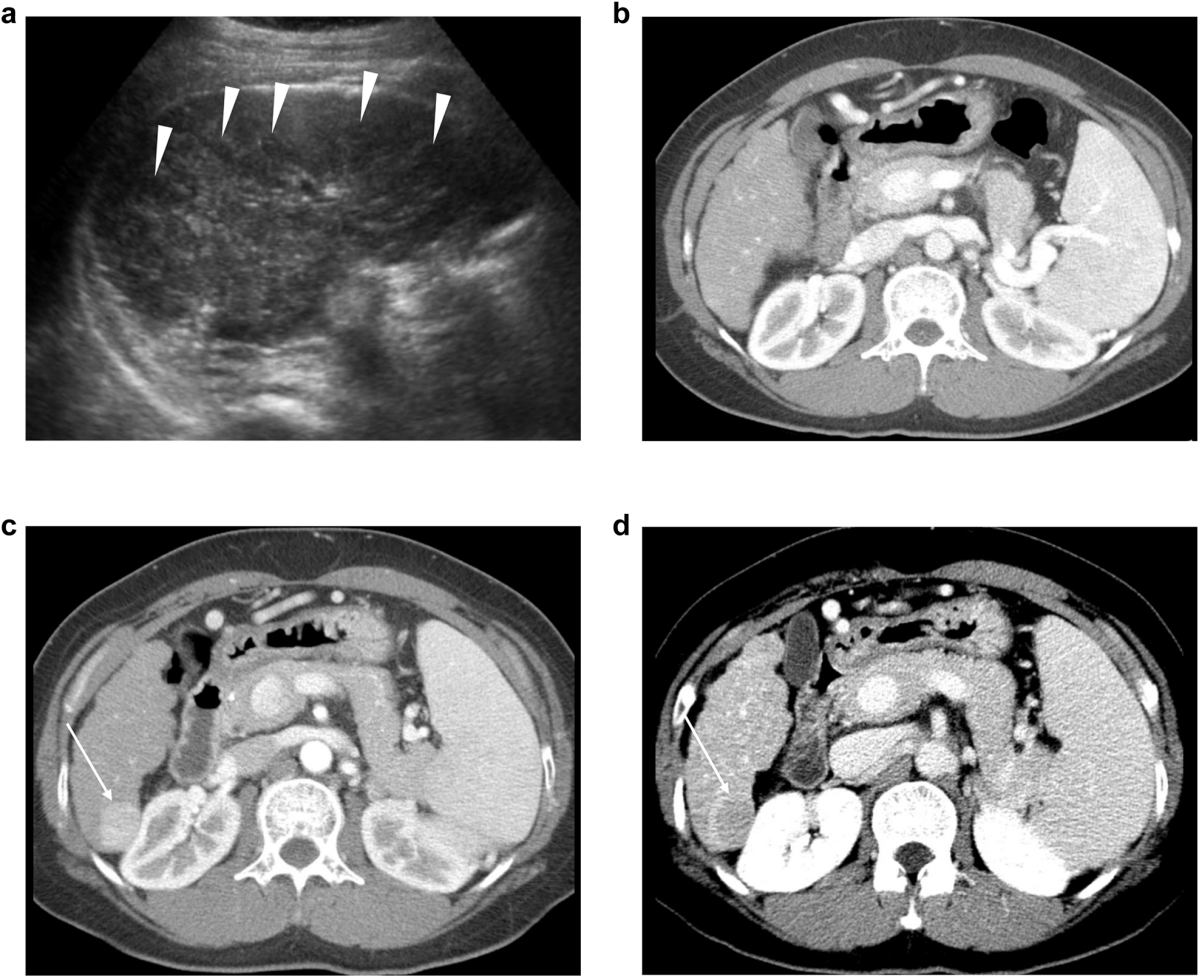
A 49-year-old non-diabetic woman with hepatitis B deemed to be high-risk by SELECT (a score of −1.93). a An index ultrasound (US) image shows cirrhotic liver parenchyma and multiple hypo- and hyperechoic cirrhotic nodules (arrowheads). On 5-month follow-up, liver CT confirms the absence of hepatocellular carcinoma (HCC) at index US (b). The patient developed HCC at an 11-month follow-up, which shows arterial phase hyperenhancement (c) and delayed washout (d, arrows). While aMAP (a score of 68.49) also classified the patients as high-risk, the other models of THRI (236), ADRESS-HCC (4.64), Velazquez score (1.66), and mPAGE-B (10) failed to classify the patients as high-risk
